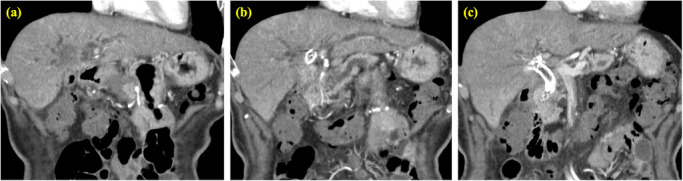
Contrast‐enhanced computed tomography before endoscopic ultrasound‐guided hepaticogastrostomy (EUS‐HGS). (a) A tumor extending from the hepatic hilum to the right hepatic lobe is observed. (b, c) Dilatation of the left intrahepatic bile duct was evident. No ascites was present, and the upper gastric body along the lesser curvature was closely apposed to the liver.
